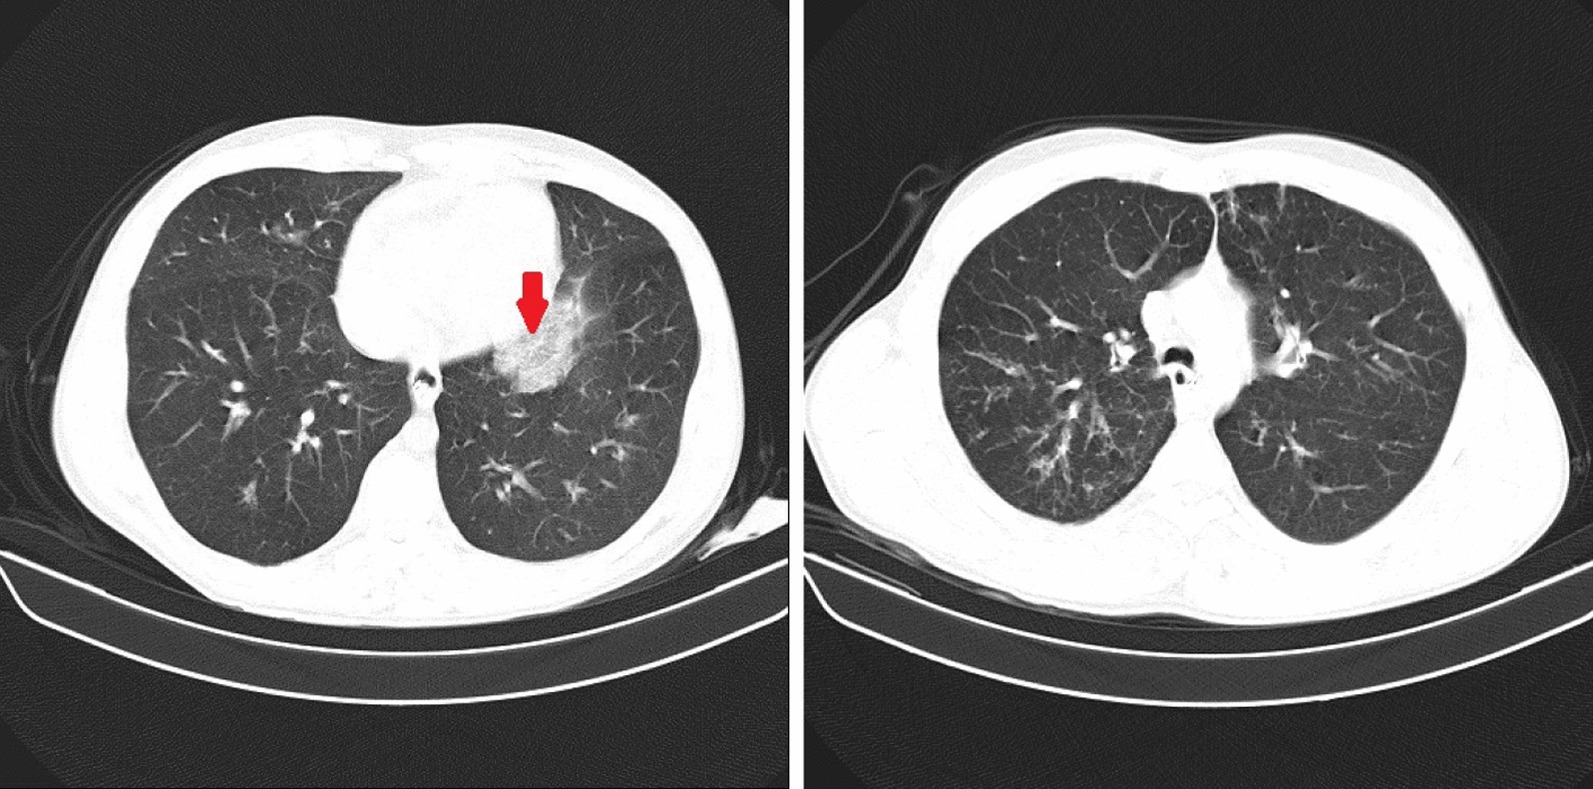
Chest CT showed bilateral peripheral and peribronchovascular patchy ground glass opacities (arrow) in both lungs with tree-in-bud appearance
